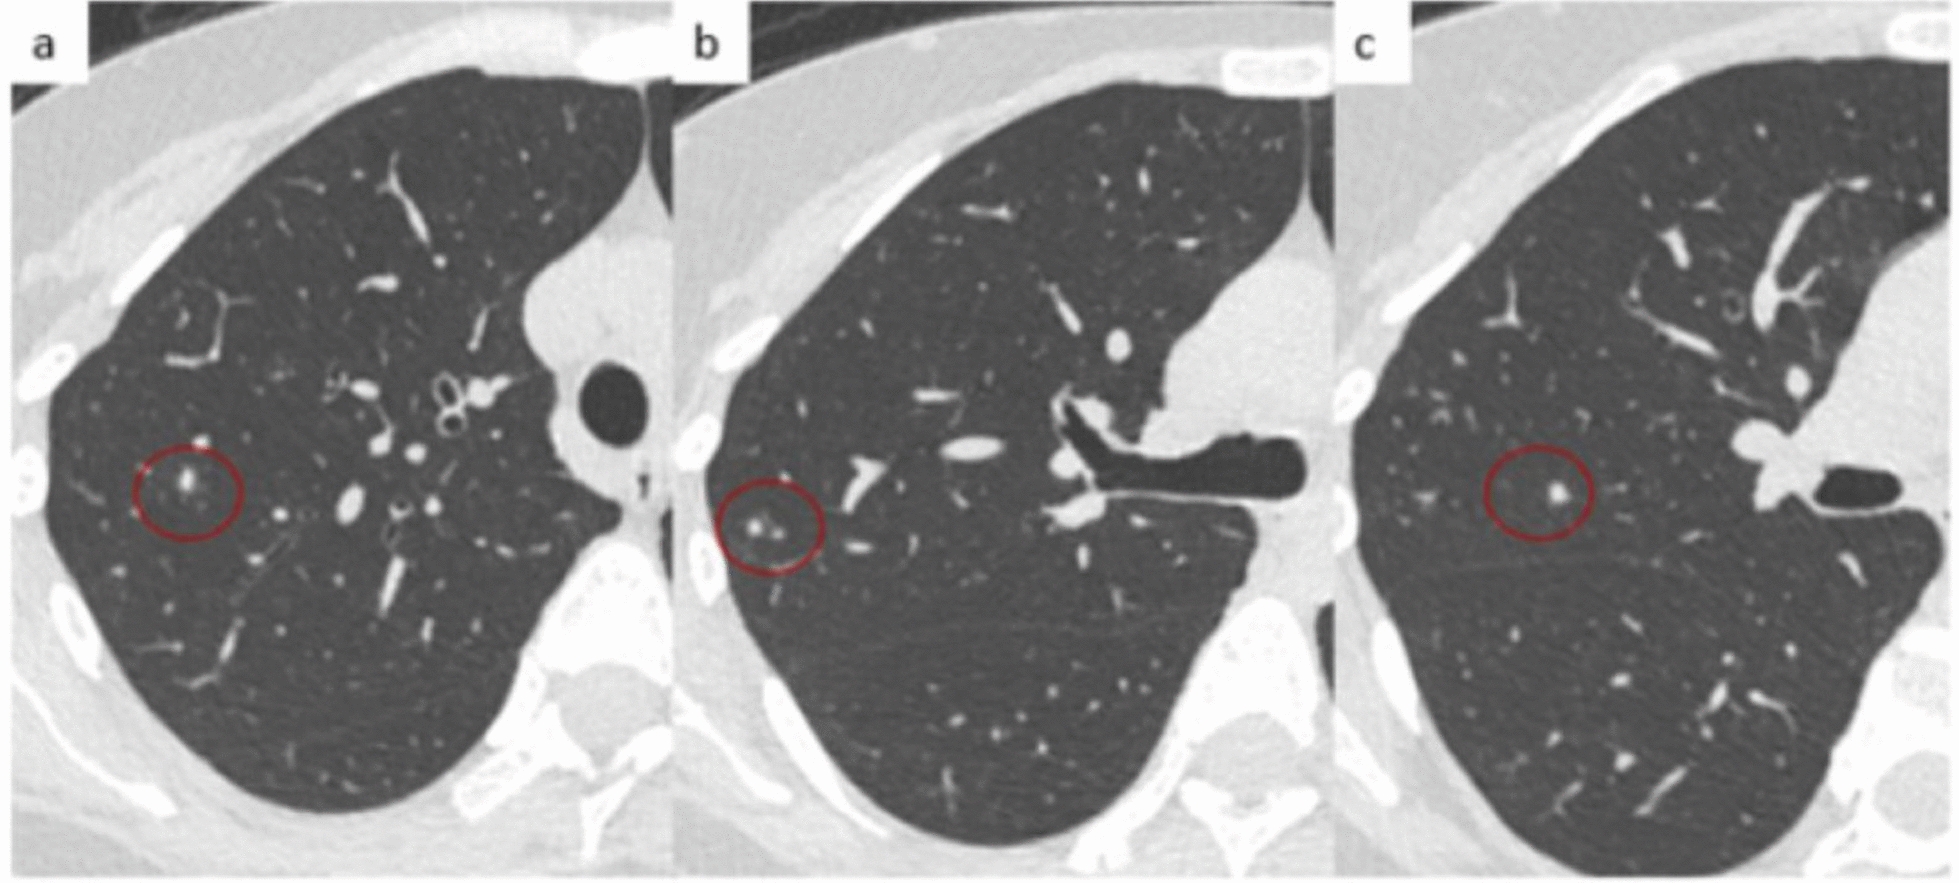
Computed tomography (CT) at diagnosis before starting therapy: the non-contrast CT scan shows the presence of solid, non-calcified nodules in the dorsal segment of the right upper lobe, without evidence of cavitation or surrounding ground-glass opacity
